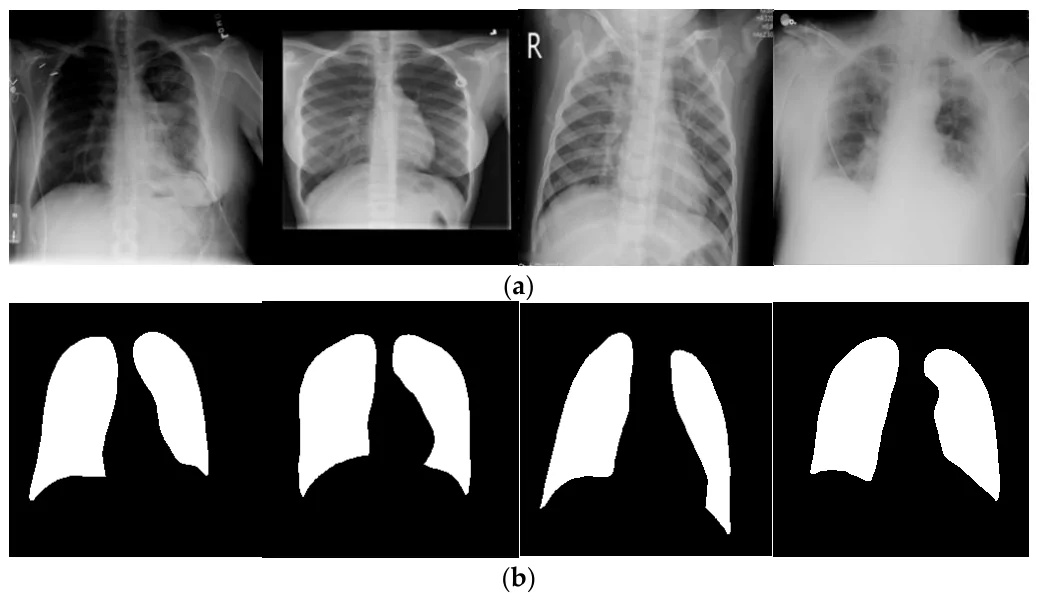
Representative images [15]. The columns show Normal, COVID-19, Viral Pneumonia, and Lung Opacity from left to right. (a) X-ray images; (b) corresponding lung masks.
